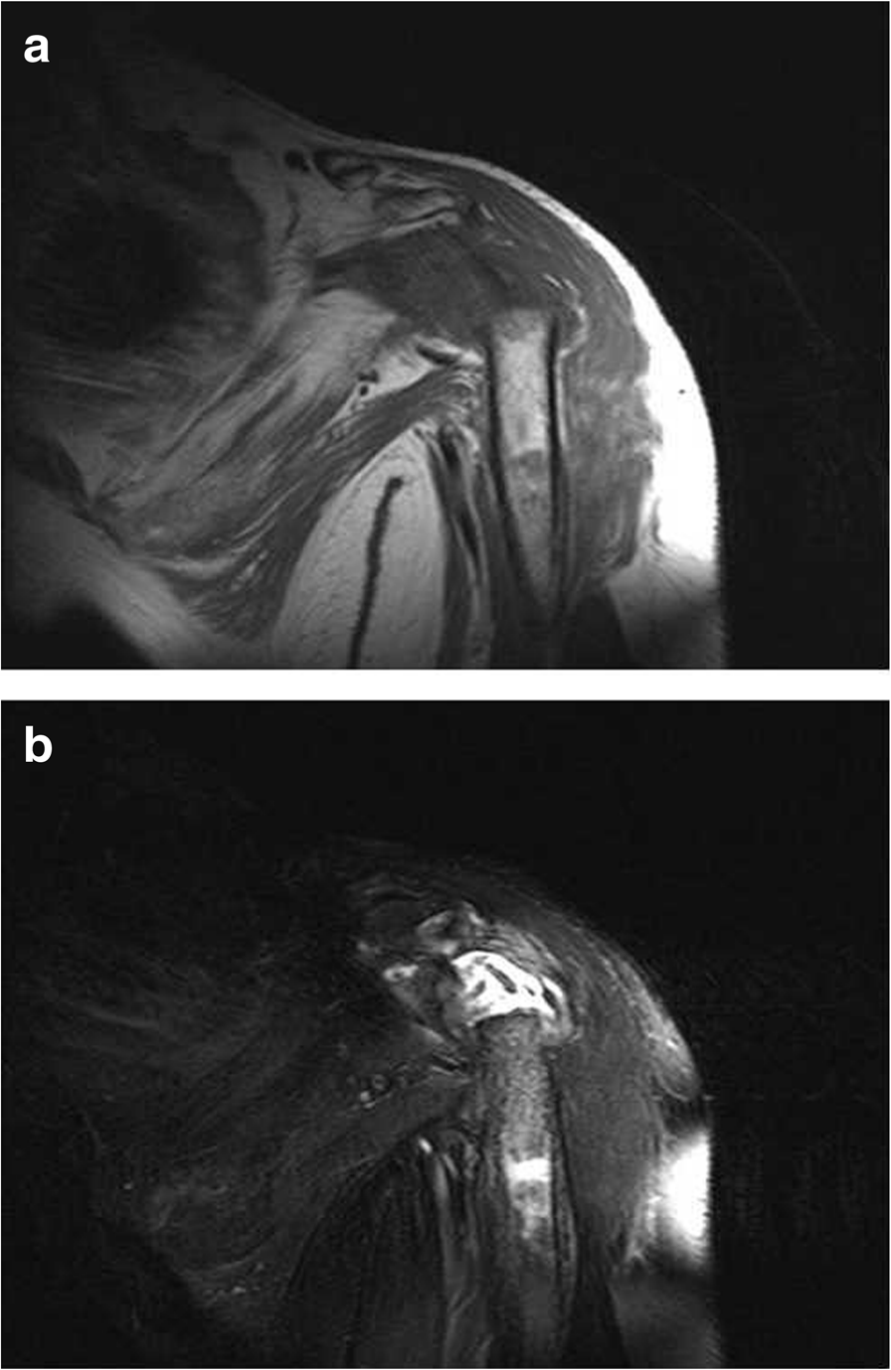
Magnetic resonance imaging. a Hypointense signal on T1-weighted images. b Areas of isointensity and hyperintensity on the T2-weighted image
